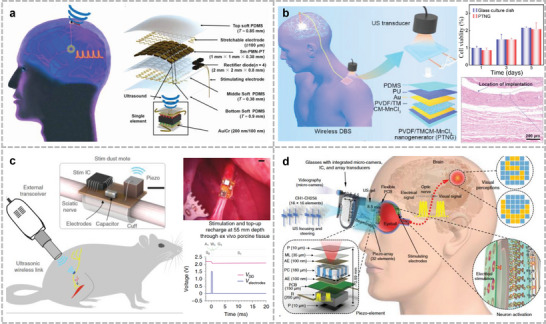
Implantable neural therapy and recording device stimulating through the ultrasonic method. a) Flexible piezoelectric energy harvester for deep brain electrical stimulation based on SM‐PMN‐PT single crystals converts collected acoustic energy into electrical stimulation. Reproduced with permission.^[^
